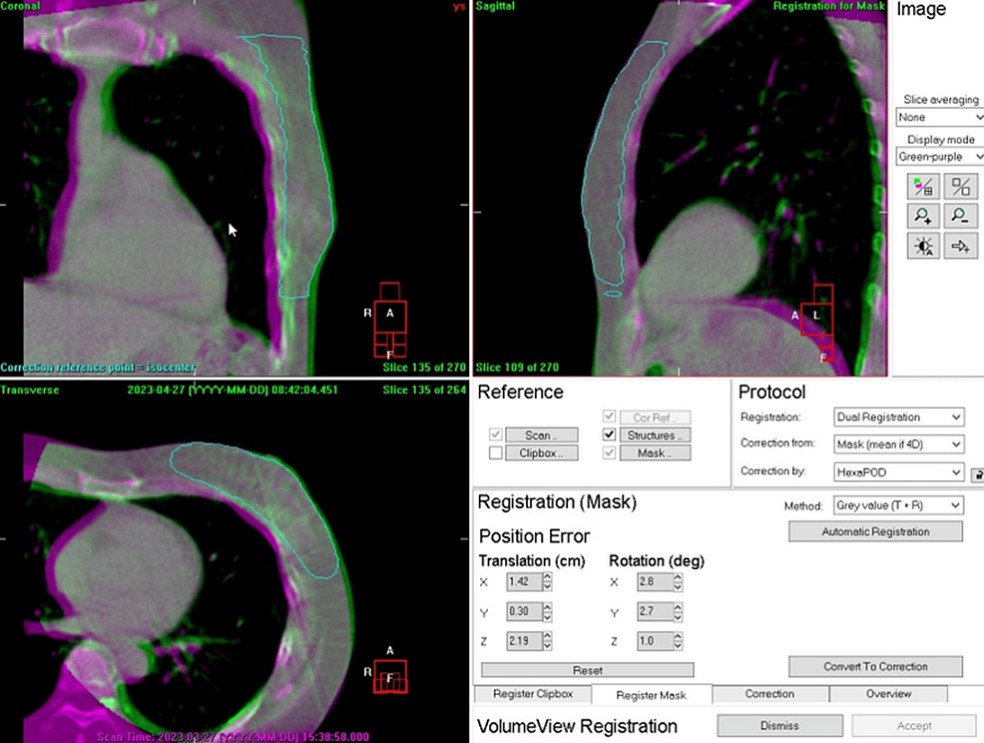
Second calculation step. Heart registration between the planning CT and cone-beam CT was performed by gray value matching inside a mask region corresponding to the heart structure defined in the plan. A subsequent manual adjustment was always conducted to match the heart surfaces proximal to the chest walls, as shown in the figure, taking into account the heart surface that may receive the highest dose.
